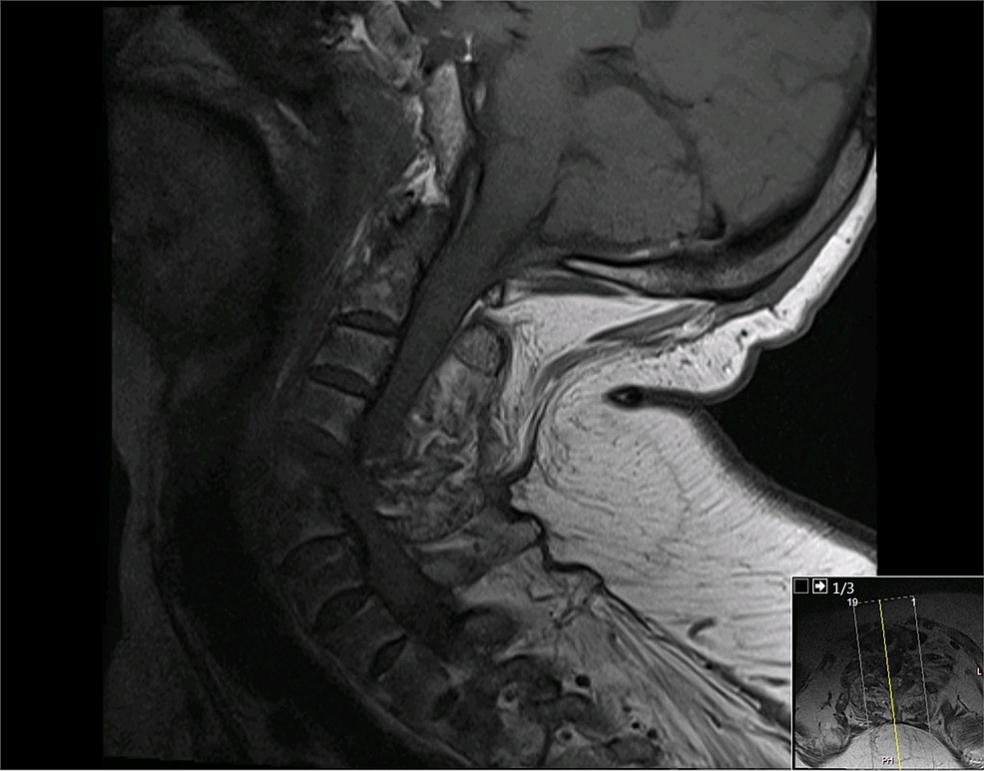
Cervical spine MRI in the sagittal T1-weighted sequence revealing a disruption injury at the C4-5 disk site and spinal cord compression
